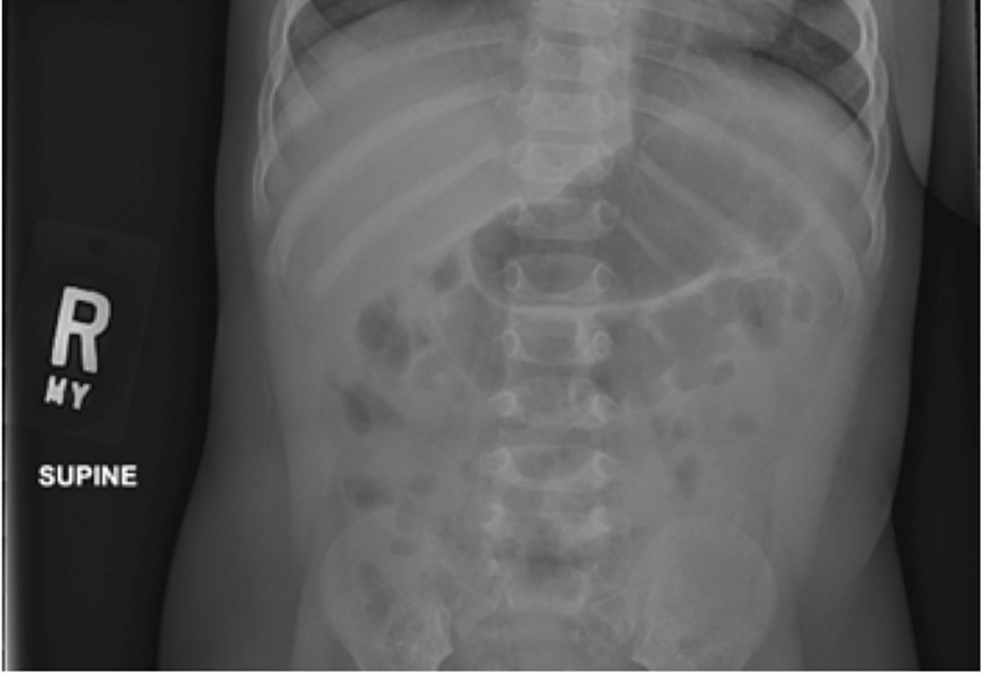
X-ray of the abdomen - first view
